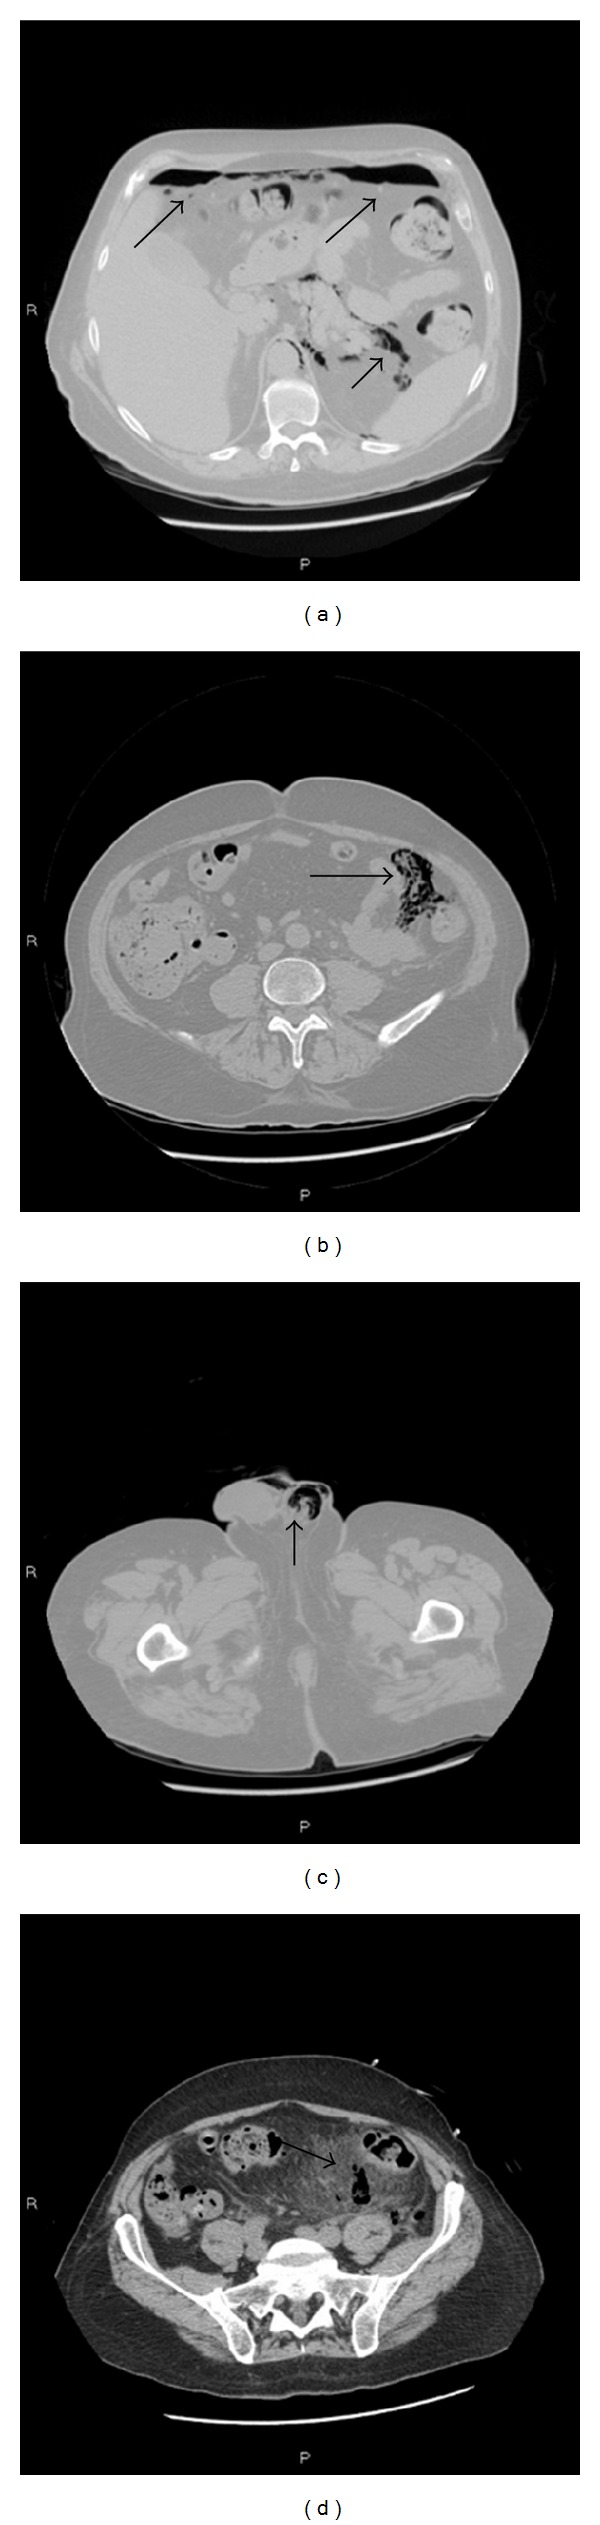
The first abdomen CT scan revealed free gas in correspondence of anterior subdiaphragmatic region (a) and left parietocolic shower (b). Gas was visualized within the scrotal sac (pneumoscrotum) (c). Peritoneal fat stranding and inflammatory changes are observed (d) (black arrows).
